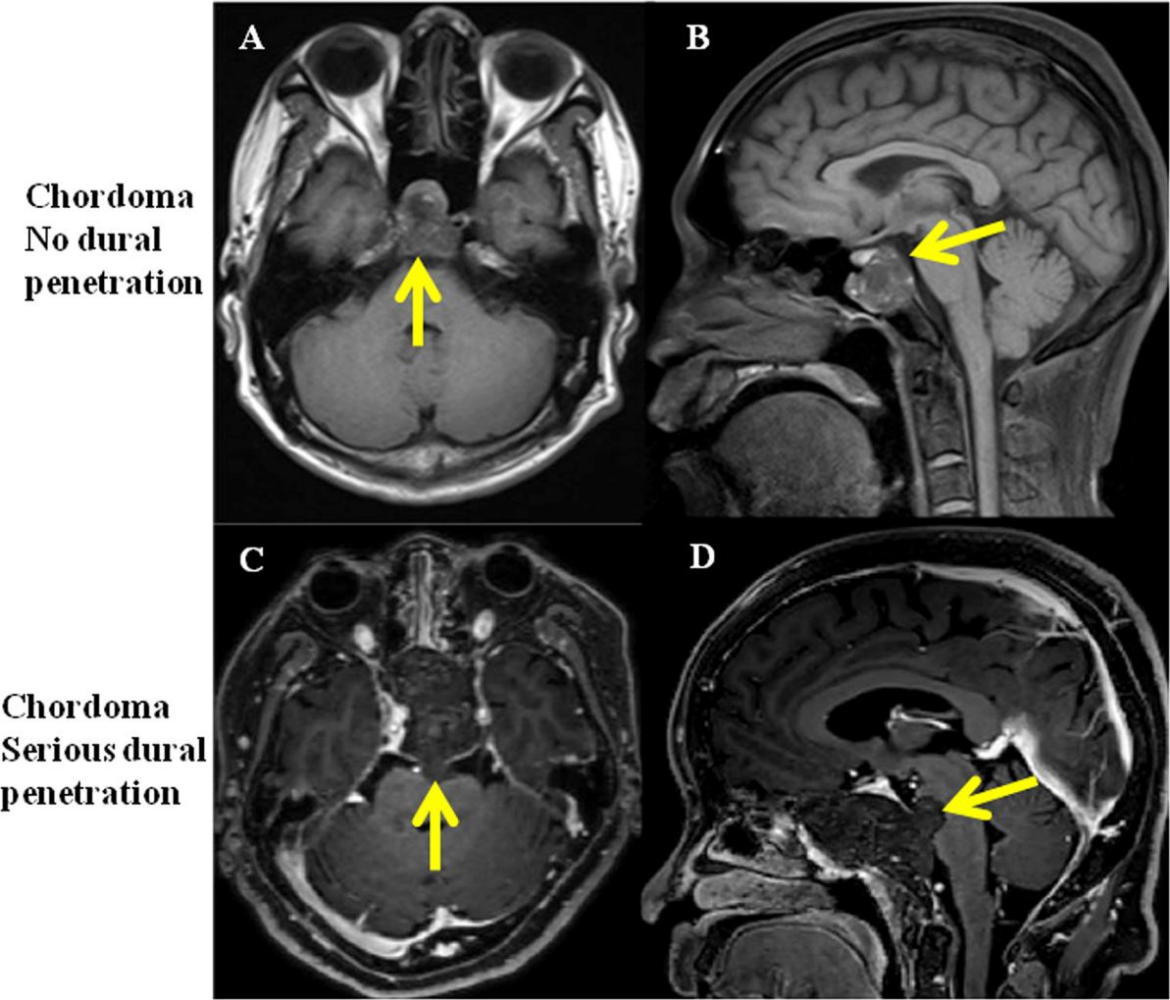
MRI of clival chordoma patients with no dural penetration and with serious dural penetration. (A, B) Same chordoma patient with no dural penetration, yellow arrows show the integrity of dura mater; (C, D) Same chordoma patient with serious dural penetration, yellow arrows show tumor penetrate the dura mater and invade into intracranial space.
